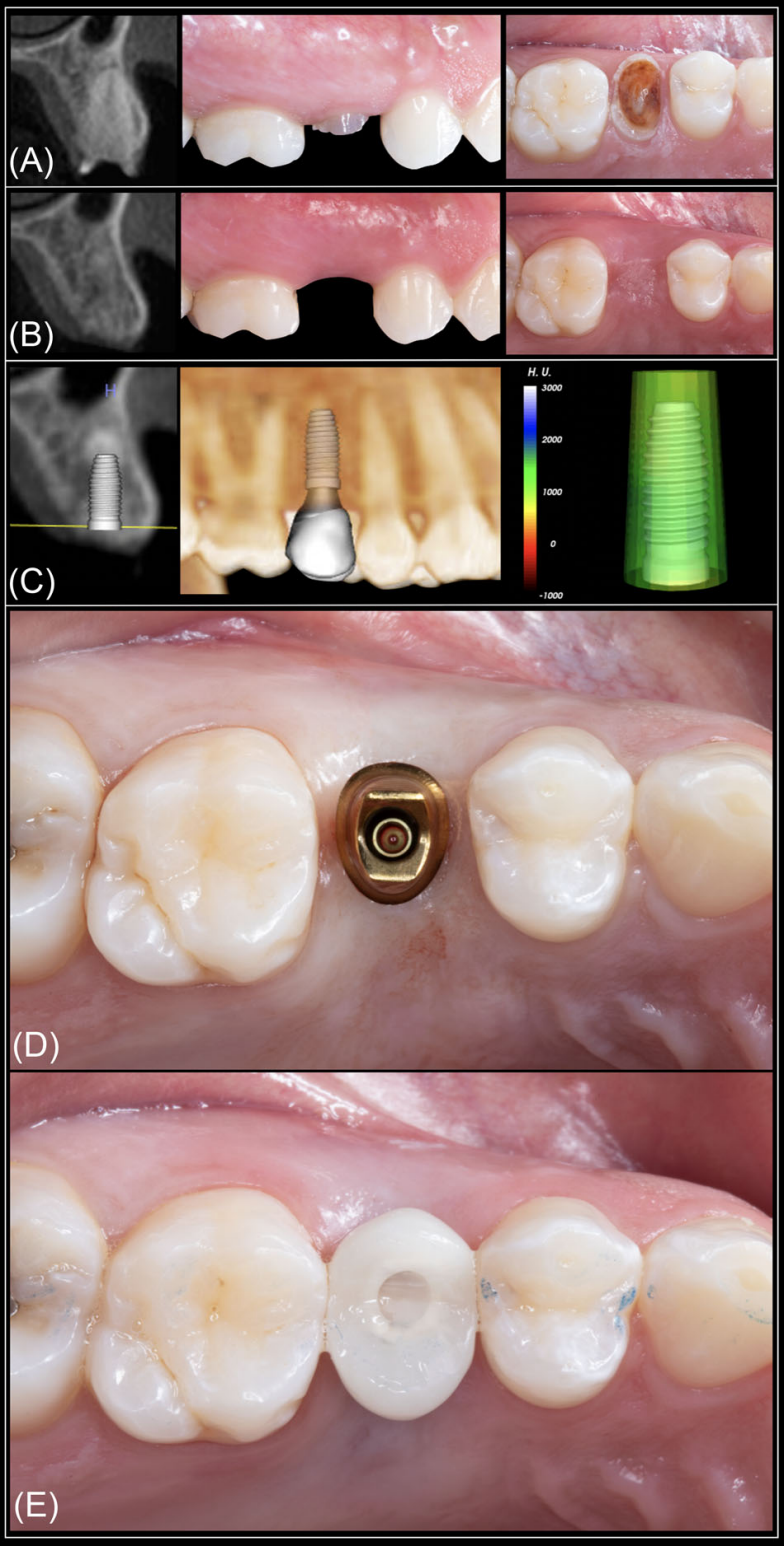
Representative case of a site presenting a thick bone phenotype. Radiographic and clinical aspect at baseline (A) and after ≈ 6 months of healing (B). After virtual implant placement, it was determined with a high degree of certainty that no additional bone augmentation would be necessary (C). Static computer‐aided implant placement was planned and executed (D). Primary stability was achieved, and a provisional custom‐made implant‐supported restoration was delivered (E). Case restored by Dr. Christopher Barwacz, Department of Family Dentistry, University of Iowa College of Dentistry
